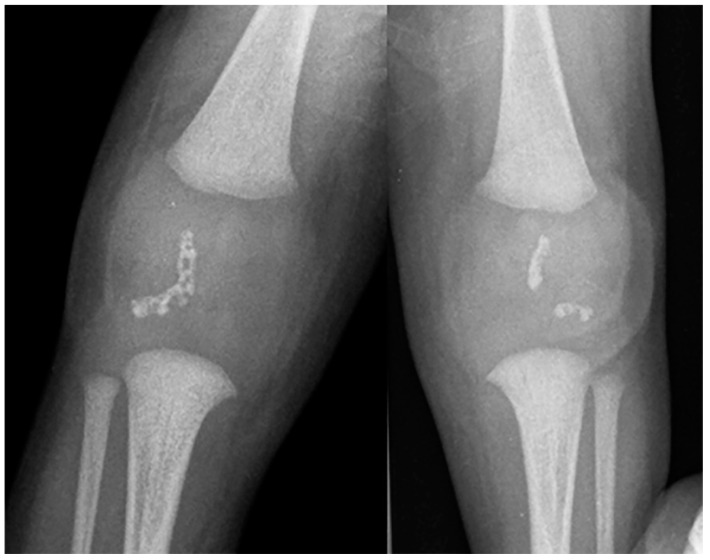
Frontal radiography of both knees in a 7-month-old male shows the characteristic crescent-shaped stippled chondral calcifications compatible with Zellweger syndrome.
